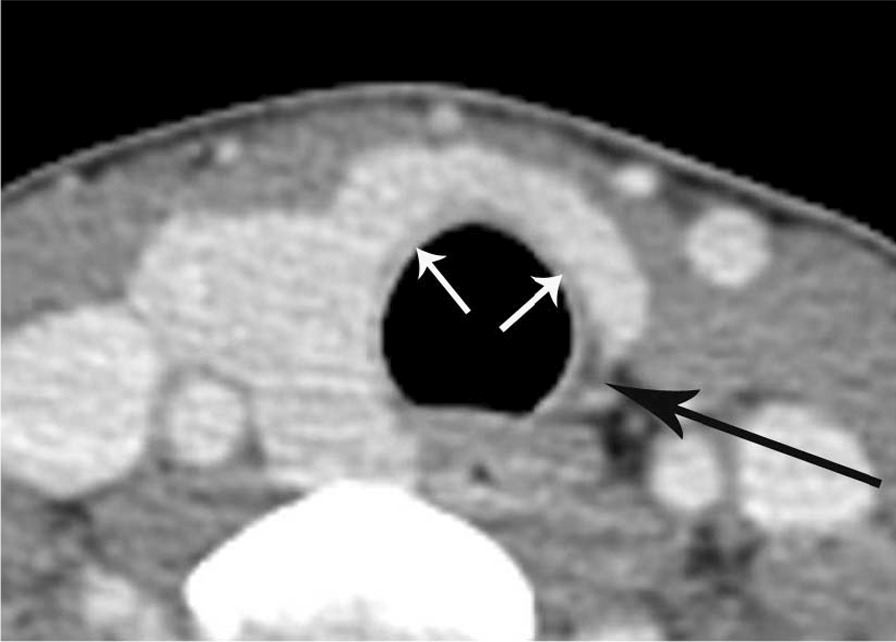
A 42-year-old woman with left thyroid hemiagenesis (case 1). Axial contrast-enhanced CT scan shows the presence of isthmus (short white arrows) and sharp edge of medial end of remnant thyroid gland (arrow). The angle of the medial end of remnant thyroid gland was measured as + 108°
